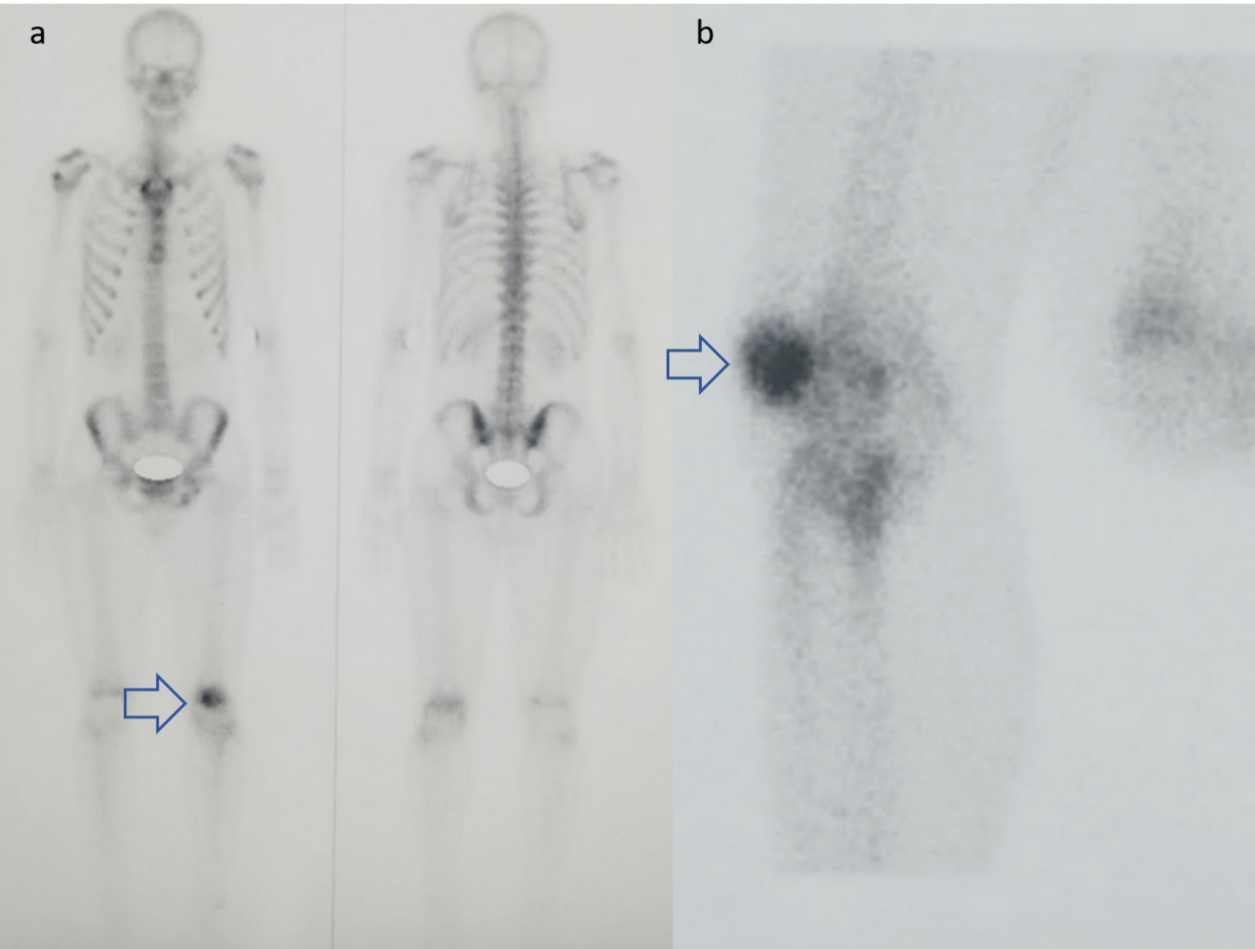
Bone scintigraphy a. Total body coronal view, b. Patella sagittal view Blue arrows: increased signal uptake on the patella
